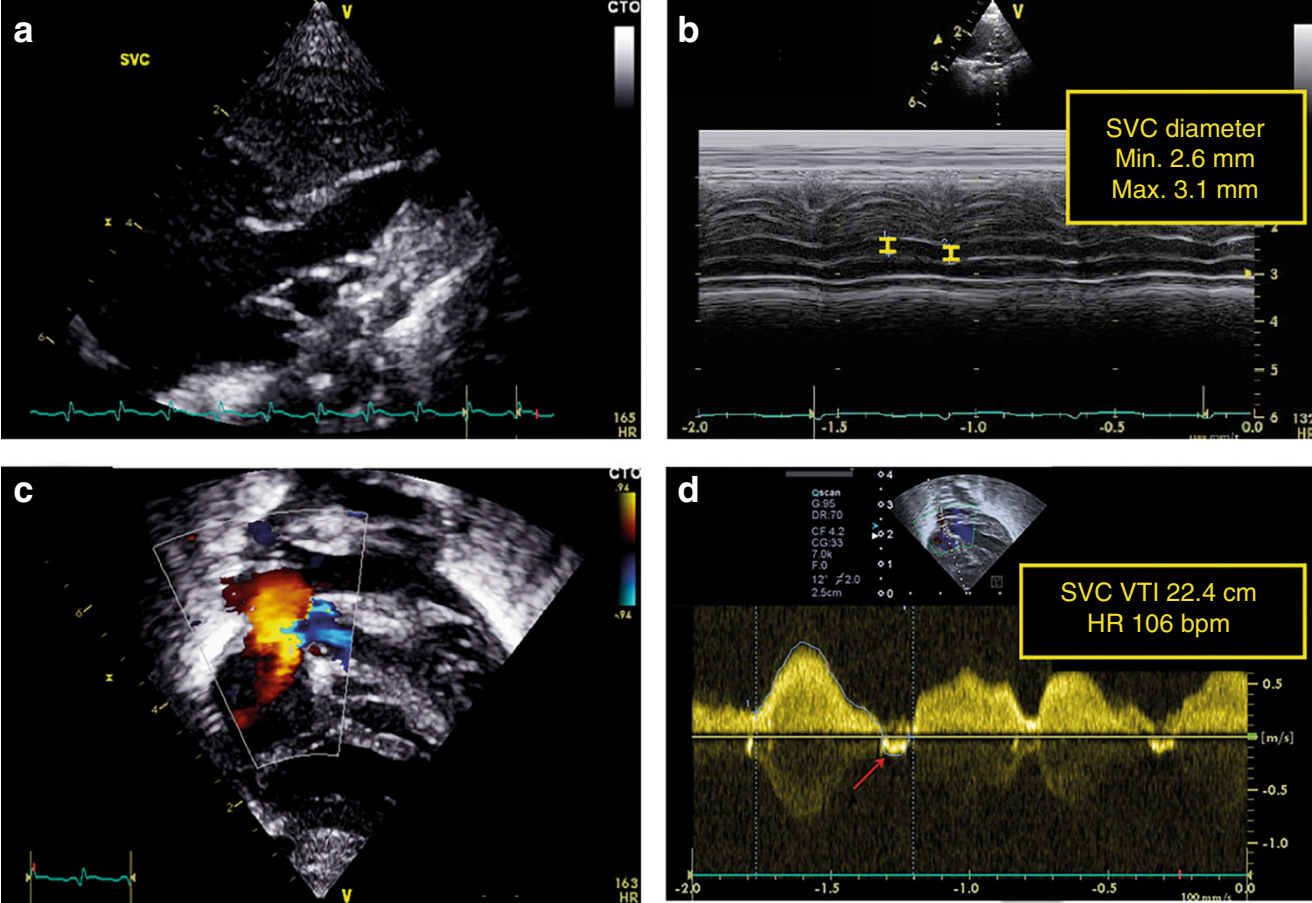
Assessment of superior vena cava (SVC) flow. The SVC diameter is measured at the entrance to the right atrium from the modified parasternal long-axis view (a). Both minimum and maximum diameters are measured through the heart cycle. Three to five consecutive heart cycles are analyzed with the average regarded as the mean SVC diameter (b). The subcostal view is used to assess SVC flow velocity (c). It is advised to average VTI tracings from eight to ten heart cycles to minimize variations secondary to respiration. Negative blood flow velocities (A-wave and sometimes a late-systolic negative wave) should be subtracted for a reliable SVC flow estimation (d)
